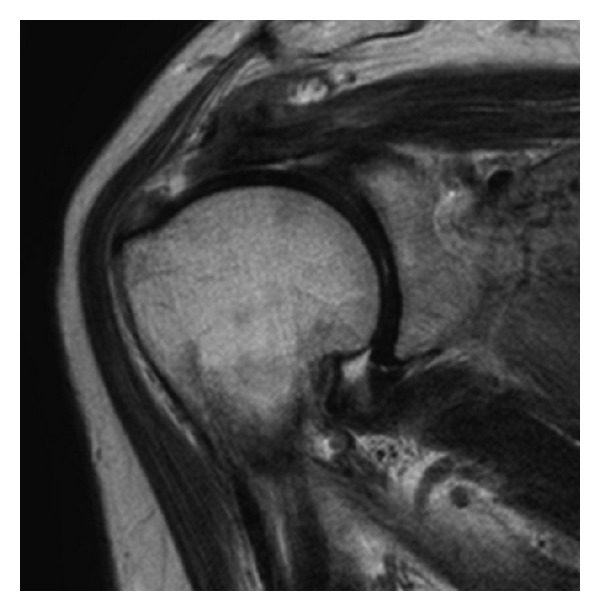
T2-weighted magnetic resonance image of the right shoulder. Tear of the supraspinatus tendon is noted.
